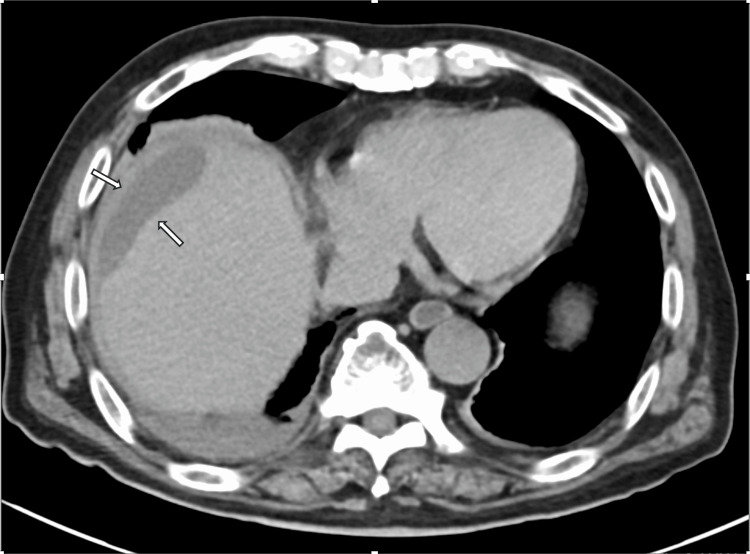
Non-contrast abdominal computed tomography showing gallbladder distension and pericholecystic fat stranding. Arrows indicate a subcapsular low-attenuation area in the anterior liver.
